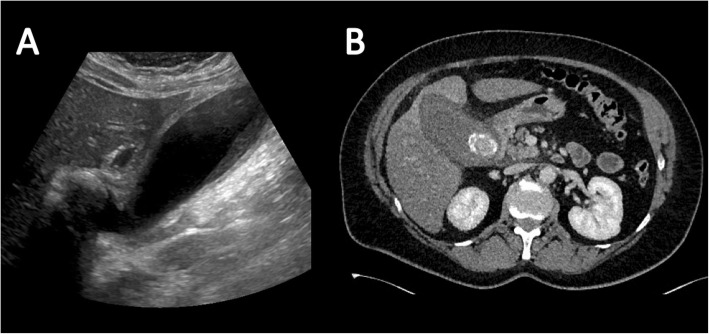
a Sagittal ultrasound of a distended gallbladder with an impacted stone in the gallbladder neck. Mild associated gallbladder wall thickening. b Axial contrast-enhanced CT of the abdomen in the same patient, again demonstrating a distended gallbladder with an impacted hyperattenuating stone in the gallbladder neck. There is subtle associated fat stranding. Findings are consistent with a gallbladder mucocoele. The gallbladder wall thickening and fat stranding are suggestive of possible empyema
